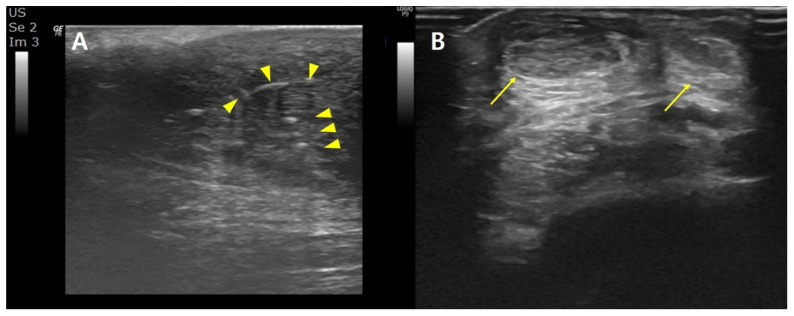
(A) Longitudinal and (B) transverse ultrasound images. Hypoechoic, heterogenous reactive cyst-like lesion around hyperechoic foci (arrow heads), indicating suture materials. Edematous and abnormally thickened tendon (arrows).
